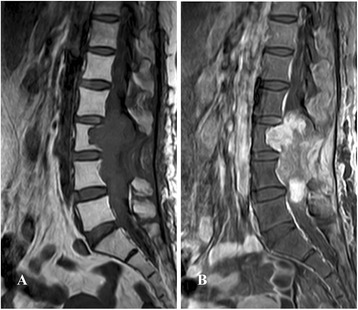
Sagittal MRI showed a large infiltrative mass in the filum terminale. a Hyperintensity MRI T2-weighted sequence; and (b) Gadolinium-enhanced MRI scan showed a large infiltrative mass with heterogeneous high signals
